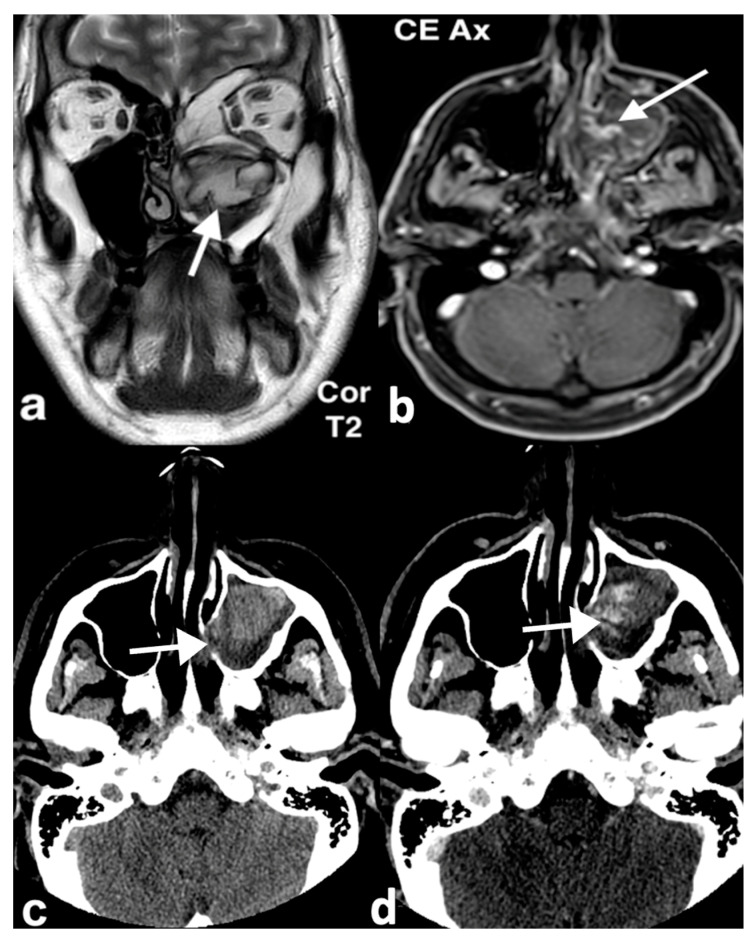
Sinonasal tract angiofibroma in a 43-year-old patient with recurrent epistaxis and left nasal obstruction, anosmia, left cheek pain, and headache for approximately one year. MRI: coronal T2 image (a) shows a lobulated mass (arrow) with peripheral low-intensity rim, centered at the left maxillary sinus and extending through an accessory ostium into the left nasal cavity, totally obstructed; on the axial fat saturation T1 after intravenous Gd image (b), the mass (arrow) shows heterogeneous enhancement with contrast media blush in the left nasal fossa, suggesting active bleeding. On axial CT (c), the mass (arrow) has a soft tissue density, showing (d) intense but heterogeneous enhancement (arrow) after intravenous iodinated contrast medium administration.
